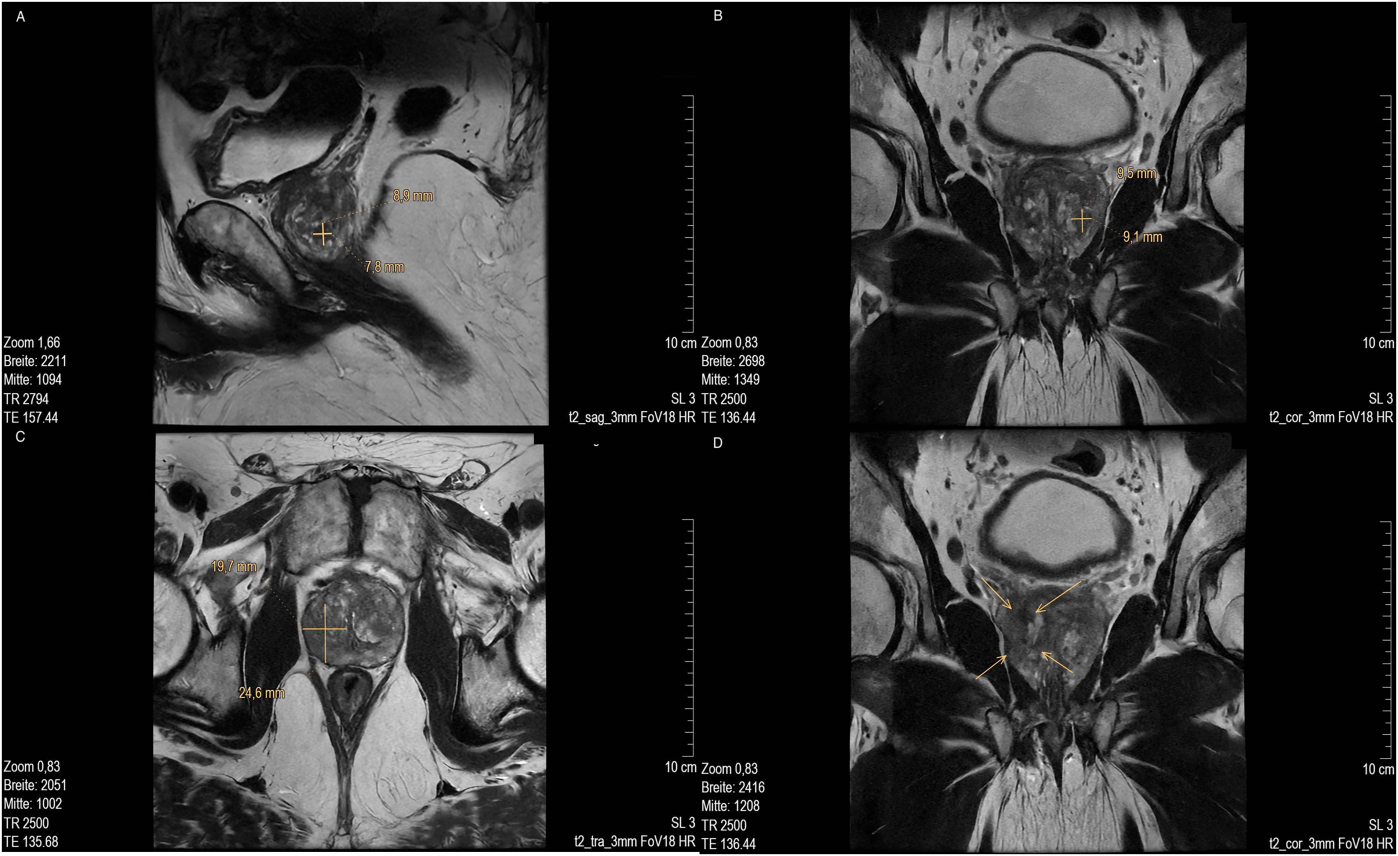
MRI scan of prostate of lesion 3. (A) Low in signal in T2 sag 3 mm FOV 18 and (marked with cross); (B) T2 cor 3 mm FOV 18. MRI of lesion 4 (marked with cross); (C) T2 tra 3 mm FOV18 (marked with cross) and (D) Low signal in T2 cor 3 mm FOV18 (marked with arrow). MR images courtesy of Dr. med. Stefan Oehm from die Radiologen, Weiterstadt, Germany. MRI, Magnetic Resonance Imaging; sag, sagittal; FOV, field of view; cor, coronal; tra, transverse
